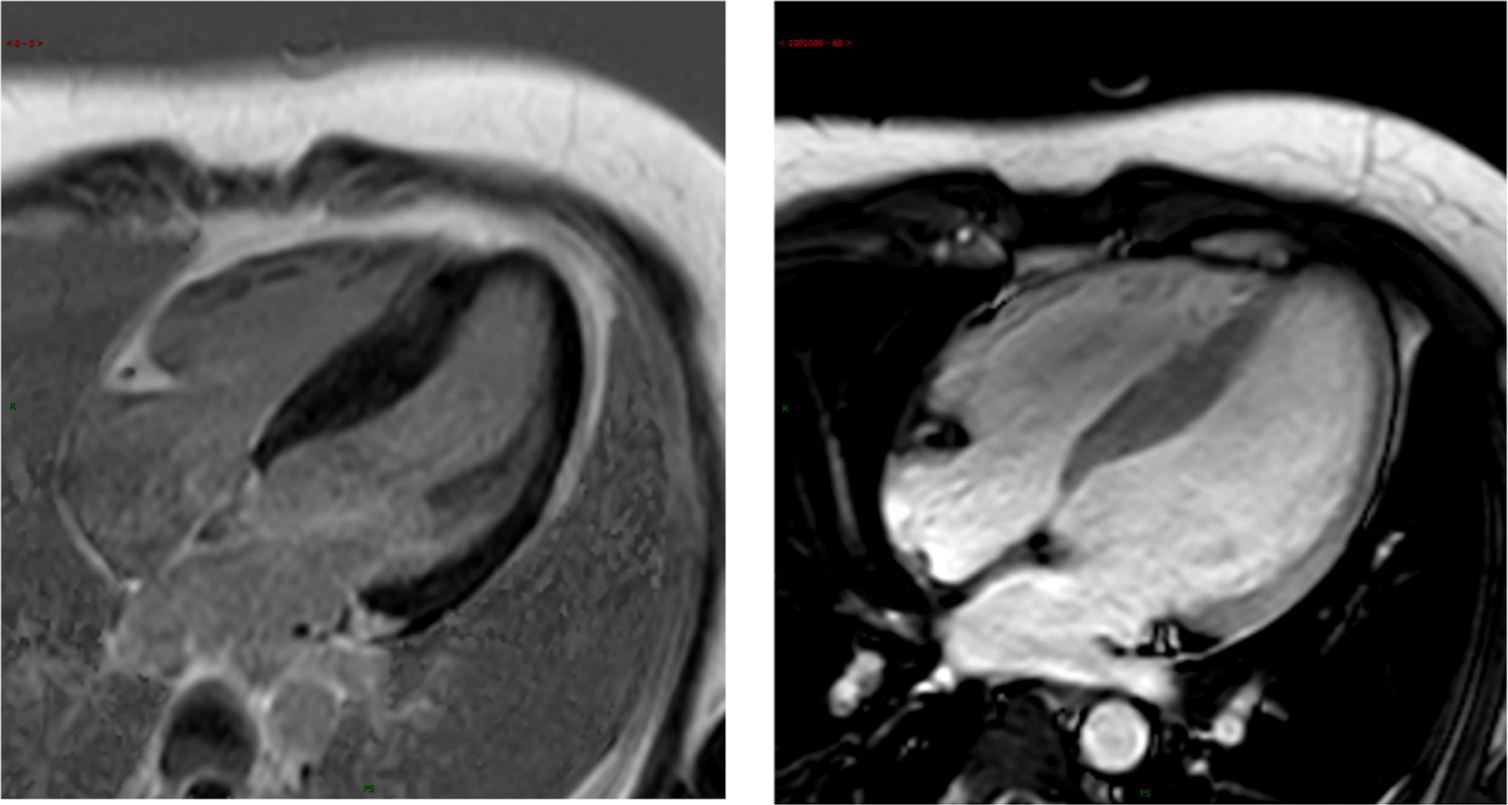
Cardiac MRI displayed normal structure and function, with a 17‐mm interventricular septum
